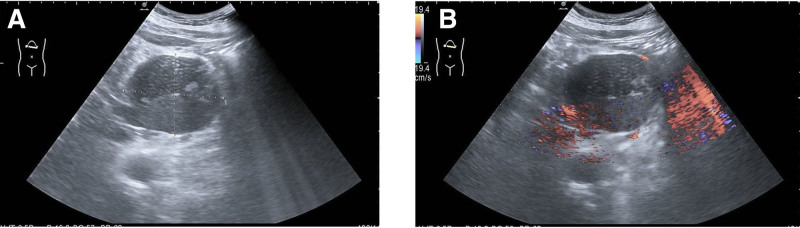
US image description. (A) Cystic mass between lower edge of left liver and upper edge of pancreas body, about 86 × 69 mm in size, clear boundary, close relationship with pancreas, poor boundary, and poor internal acoustic transmission. (B) Tumor CDFI: blood flow signals were seen in the wall of the sac. CDFI = color doppler flow imaging, US = ultrasonography.
